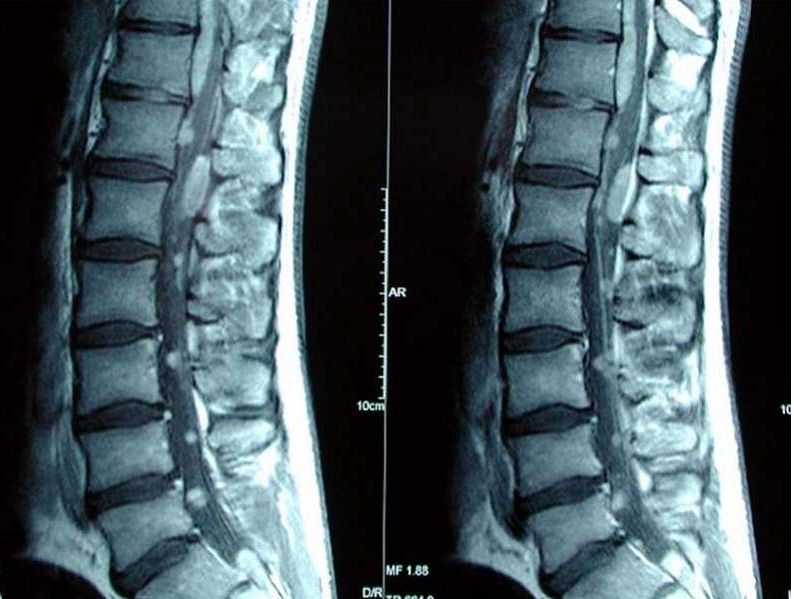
Sagittal magnetic resonance imaging (MRI) scans of the lumbo-sacral spine showing multiple ependymomatous lesions.
